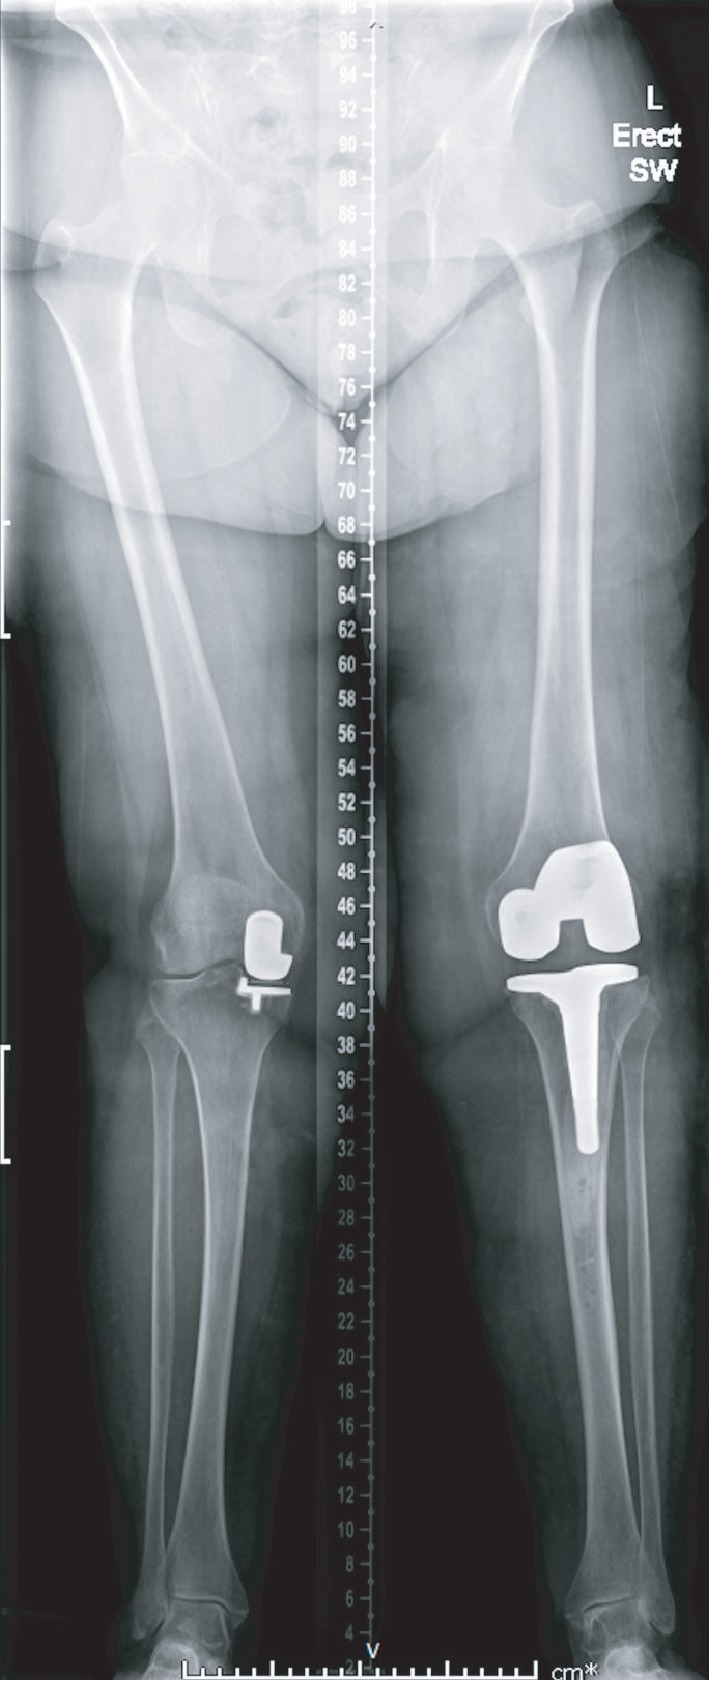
Long-leg radiographs demonstrating a medial unicompartmental knee replacement and revision left total knee replacement at six months.
